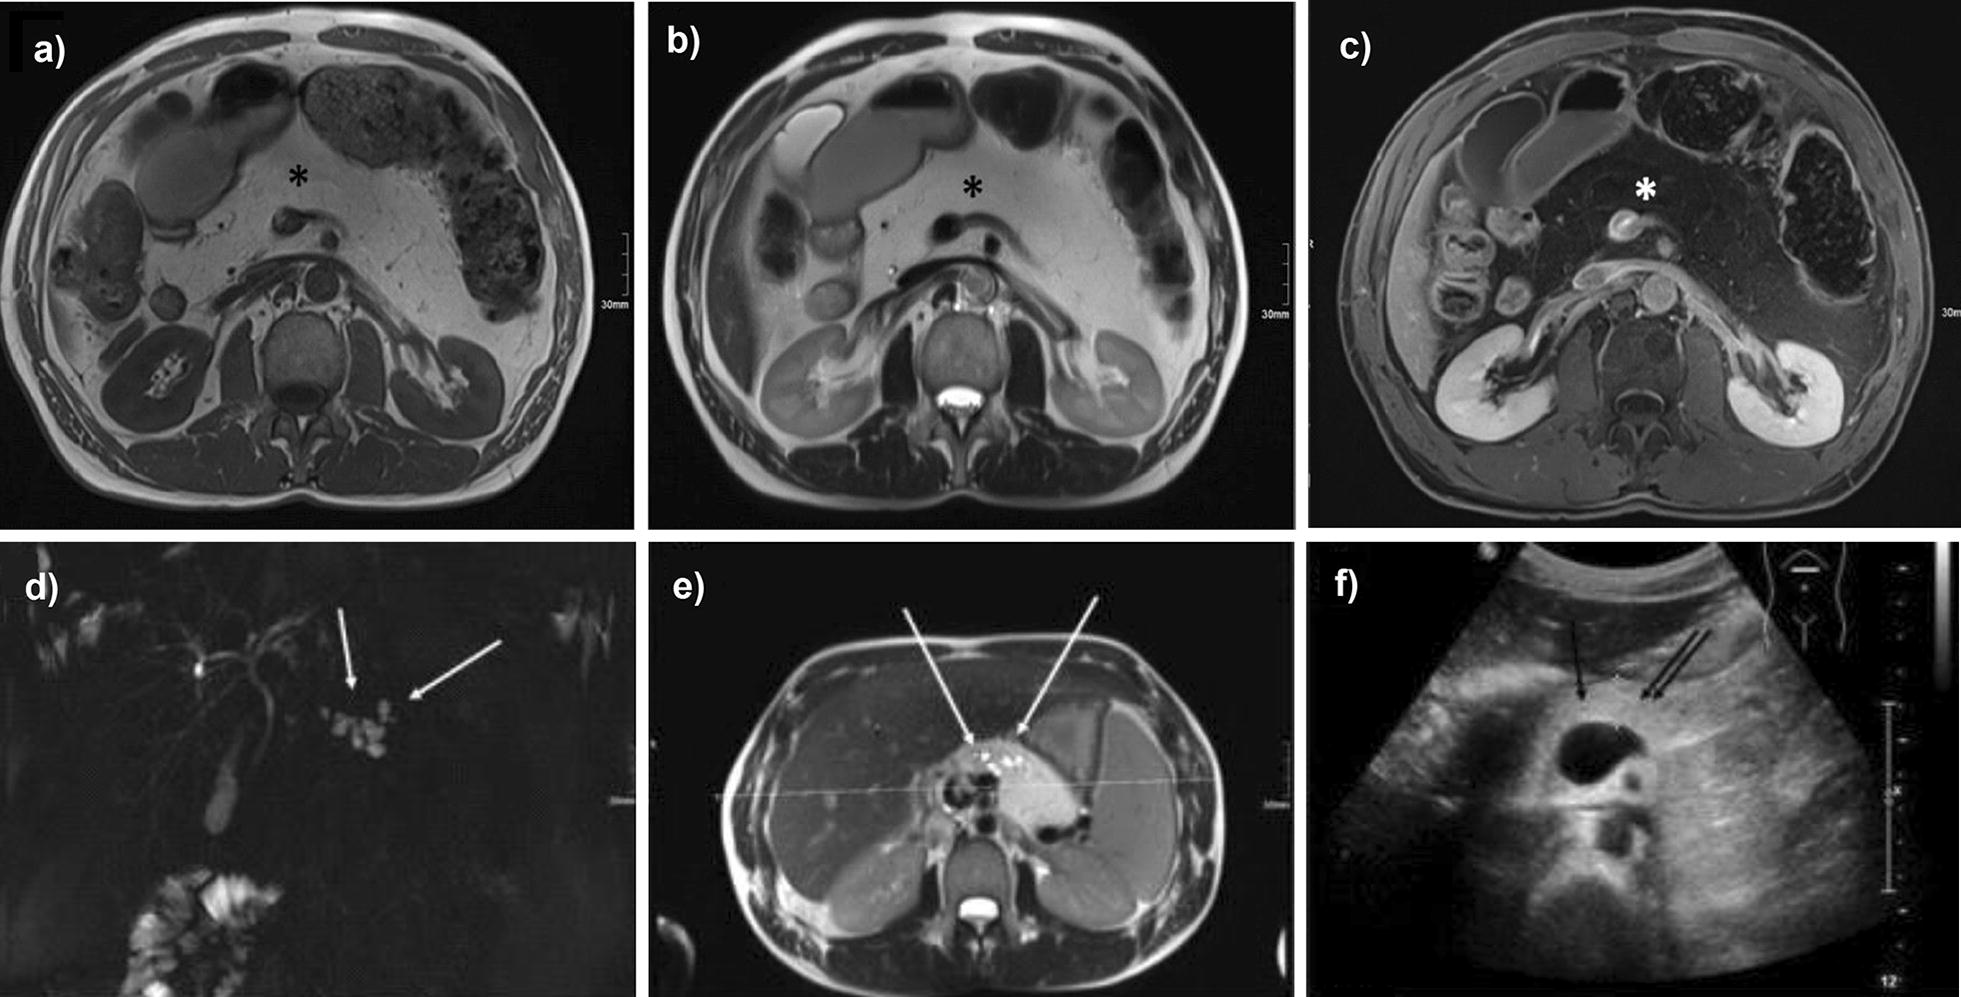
Transversal MRI images and a patient with manifold side branch cysts in pancreatic body. In all acquired sequences [T1-Flash-2D transversal native (a); T2-HASTE transversal (b); T1-Flash-2D fatsat transversal after contrast injection (c)] no residual pancreatic parenchyma can be identified. Asterixis mark empty pancreatic bed after full fatty transformation. Patient with manifold side branch cysts in pancreatic body with can be easily identified in T2-Space3D coronar MIP-MRCP sequence (d) and transversal T2-HASTE sequence (e). In B-mode sonography (f), identification of exact number, localization and contact to pancreatic duct was not possible
